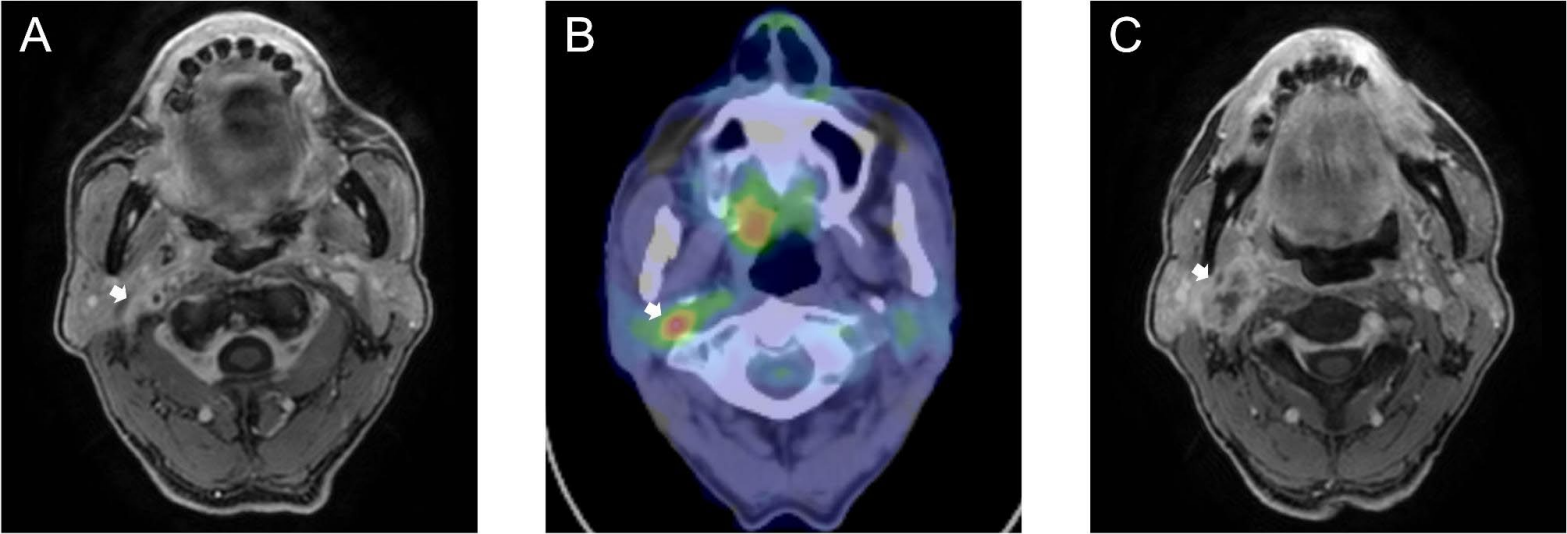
Hypopharyngeal carcinoma with ipsilateral retro-styloid lymph node metastasis (RSLNM). (A) Contrast-enhanced fat-saturated eTHRIVE and (B) [^18^ F]-fluoro-2-deoxy-D-glucose-positron emission tomography/computed tomography–fused images show RSLNM (arrow; longest diameter = 13 mm, SUV_max_ = 5.2). (C) Contrast-enhanced fat-saturated eTHRIVE images. The longest diameter of LN in the upper limit of ipsilateral level II was 25 mm (arrow)
